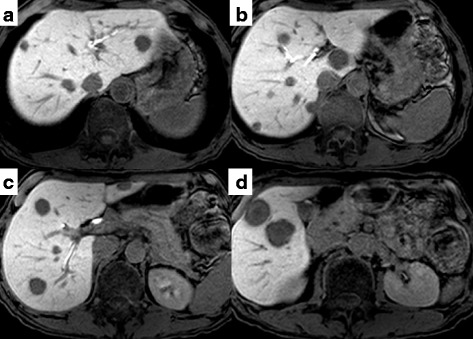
Gd-EOB-DTPA-enhanced MRI on hepato-biliary-phase findings of CRLM upon admission. a–c A large number of bilateral CRLM were detected on hepato-biliary-phase images of EOB-MRI as homogenous hypointensity. d The largest metastasis measured 2.5 cm in diameter on segment 5
